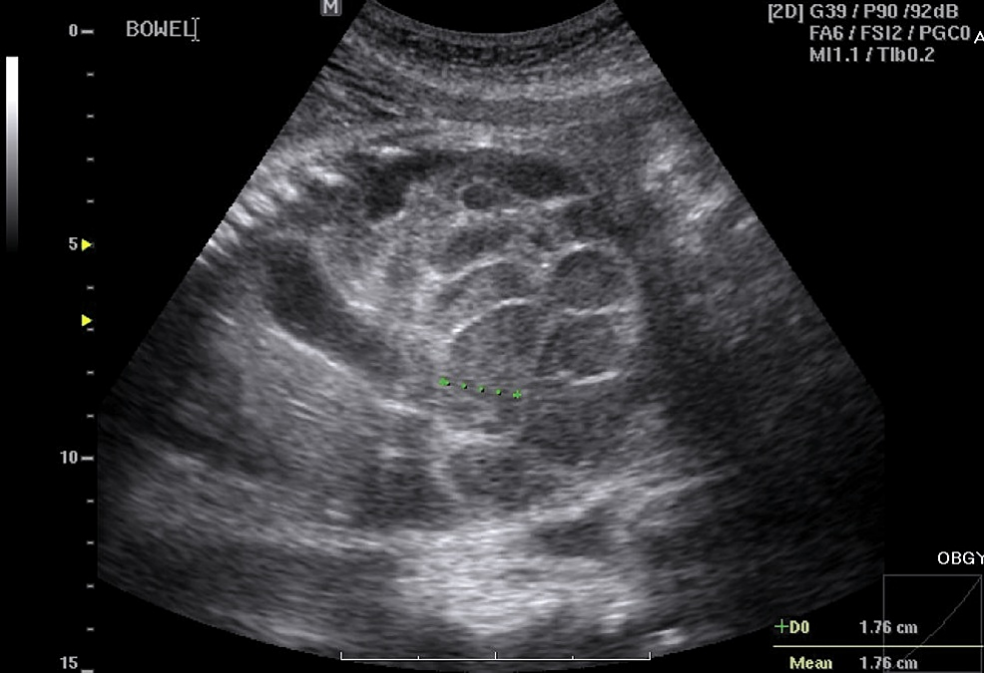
Markedly dilated intestine found by ultrasound
